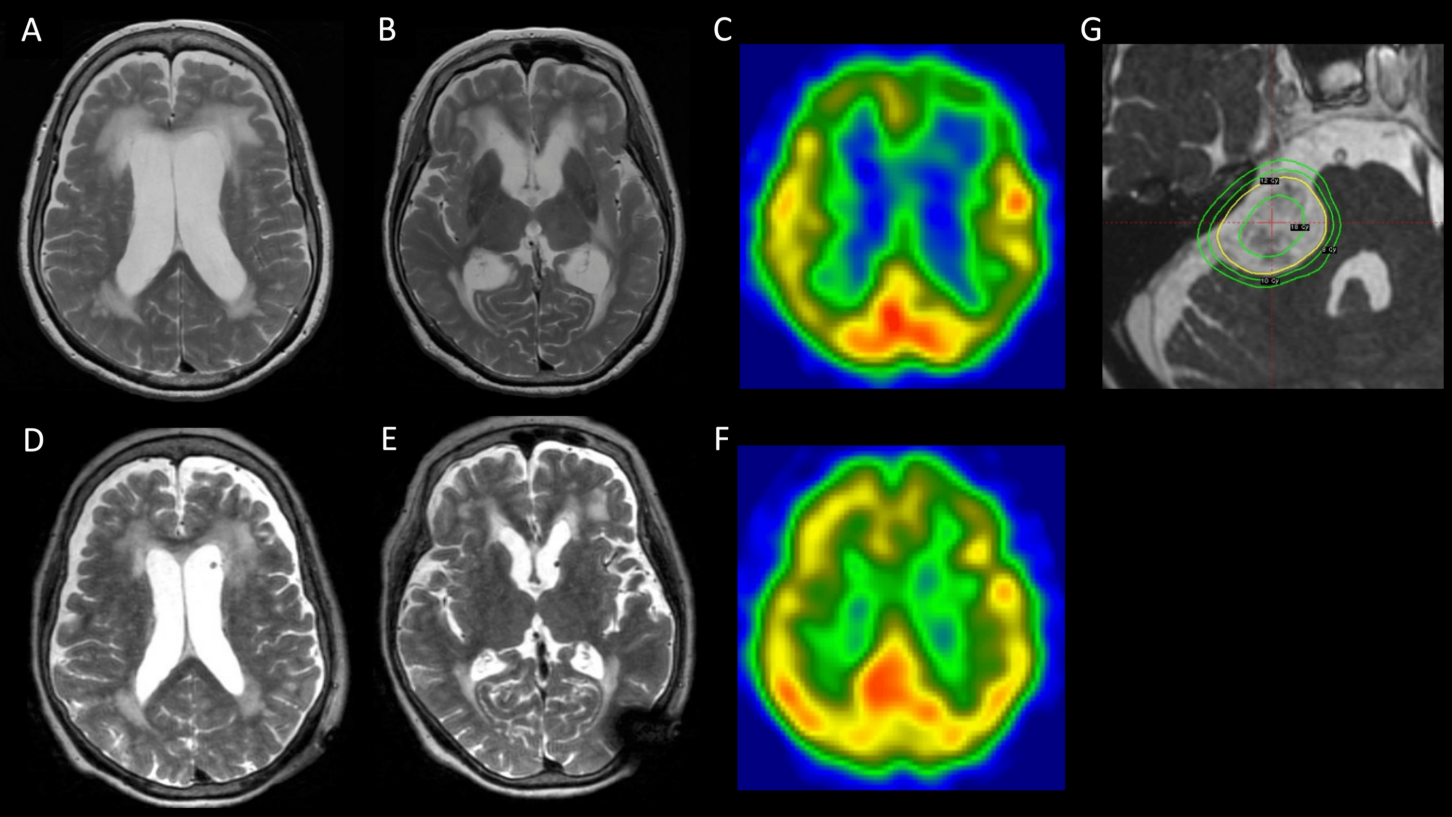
Brain MRI and 123I-IMP SPECT findings before and after ventriculoperitoneal shunting MRI: magnetic resonance imaging; ^123^I-IMP SPECT: N-isopropyl-p-^123^I-iodoamphetamine single-photon emission computed tomography; T2WI: T2-weighted imaging; SRS: stereotactic radiosurgery (A, B) Brain MRI on axial T2WI at the time of admission (Evans index, 0.34). (C) ^123^I-IMP SPECT at the time of admission shows hypoperfusion in the bilateral frontal lobes. (D, E) Brain MRI on axial T2WI seven days after ventriculoperitoneal shunting shows improved ventricular enlargement (Evans index, 0.28). (F) ^123^I-IMP SPECT 25 days after ventriculoperitoneal shunting shows improvement in bilateral frontal hypoperfusion. (G) SRS protocol using the Leksell Gamma Knife shows a marginal dose of 12 Gy and a central dose of 24 Gy
